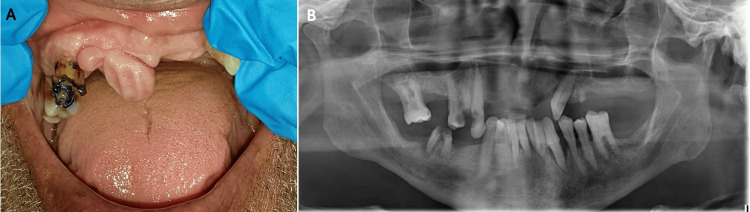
Intra-oral and radiographic examination (A) Intra-oral examination shows a well-defined solitary, bi-lobulated, firm, pedunculated exophytic lesion on the upper anterior alveolar ridge. (B) Panoramic radiograph showed no bone involvement
